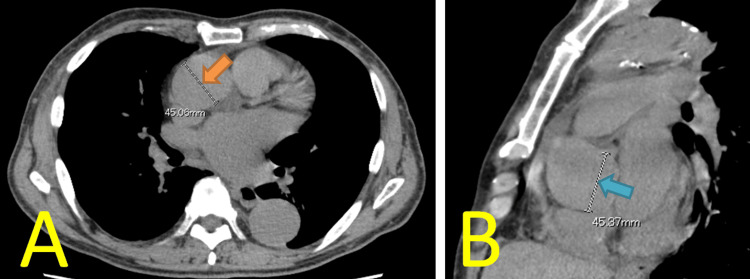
Preoperative findings of double valve replacement A. Plain computed tomography revealed that the ascending aorta was 45 mm in diameter (orange arrow, axial view). B. The sinus of Valsalva was 45 mm in diameter (blue arrow, sagittal view).
